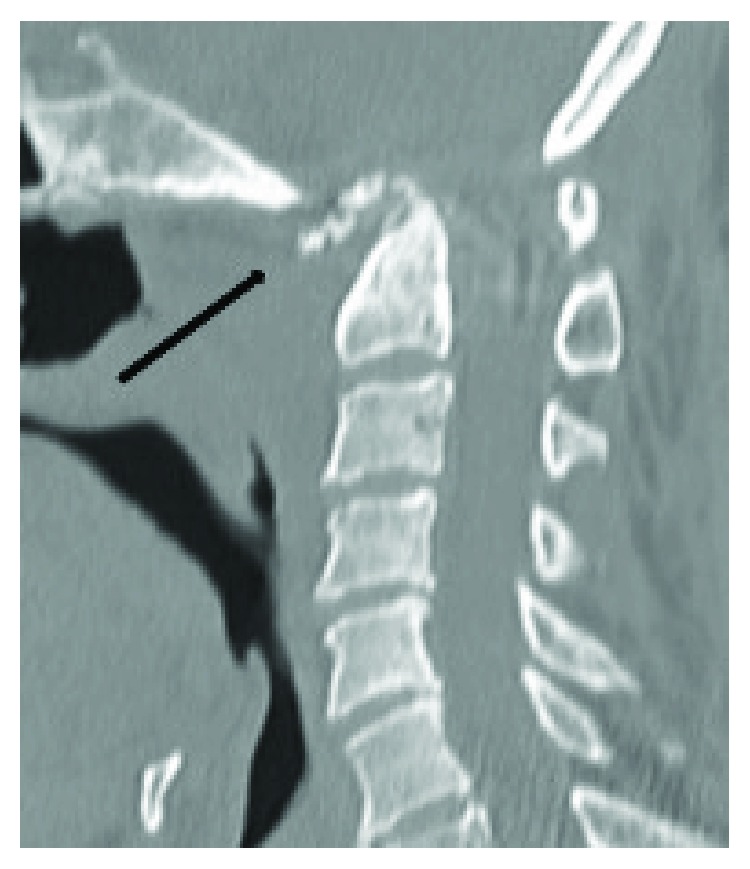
Computed tomography (CT) of the cervical spine showing erosion of anterior arch of C1 vertebra and dens (black arrow).
